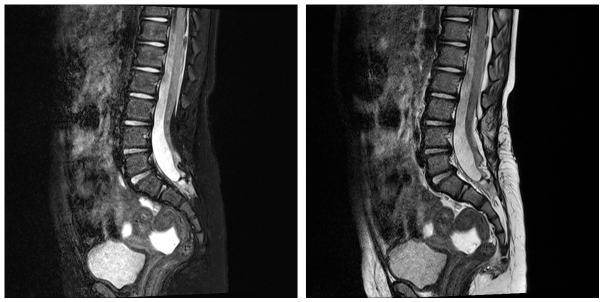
Post-operative sagittal MRI obtained one year after surgery. Left: T1-weighted image; Right: T2-weighted image. The intradural lipoma has been debulked, and the placode has been reconstructed and reduced to a tubular configuration; a capacious dural sac with a low cord–sac ratio is seen, consistent with an expansile duraplasty. No evidence of re-tethering, recurrent lipoma, or pseudomeningocele is identified.
